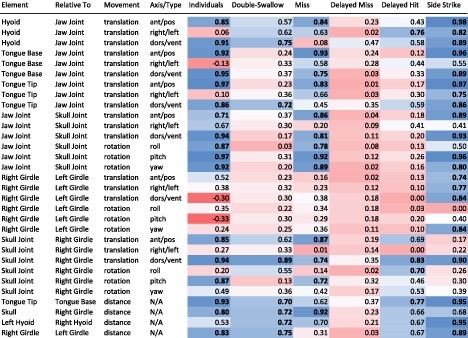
We tabulated our timing variables (e.g., maximum tongue protrusion, maximum jaw gape angle; see Supp. Table 2) for each event and converted the frame counts into duration in seconds by dividing the frame number by the frames per second (FPS) setting of our X-ray camera (250 FPS). These timing data were converted into cycle percentages by multiplying the seconds value by 101 and then dividing by the cycle duration for each event. We then calculated the mean and standard error (SE) for each of these variables for each individual for: successful, single-swallow, straight events (RM01, n = 11; RM02, *n* = 13; RM03, *n* = 12), miss events (RM01, *n* = 1; RM03, *n* = 1), double-swallow events (RM01, *n* = 1; RM03, *n* = 1), side strike events (RM01, *n* = 1; RM03, *n* = 1), delayed-swallow misses (RM03, *n* = 1), and successful, delayed-swallow events (RM01, *n* = 1).
